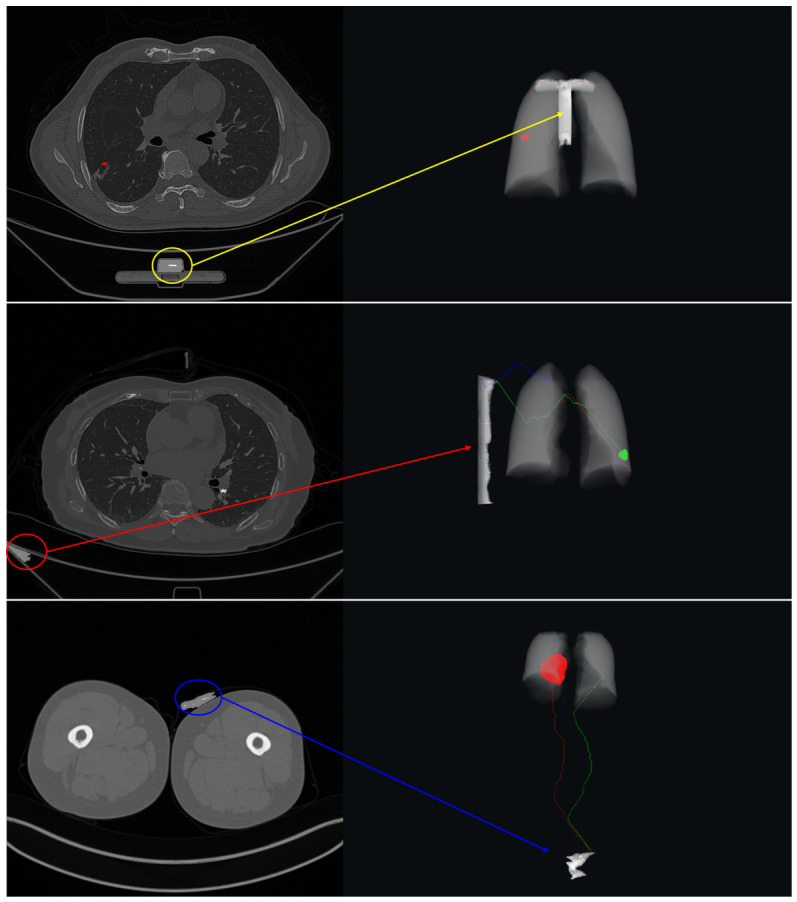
Three types of airway segmentation failure observed in the Lung-PET-CT-Dx dataset. For each row, the axial CT slice (left) and the resulting 3D path-planning output (right) are shown. (Top, yellow) Type 1: the central longitudinal air channel of a Philips scanner bed is misclassified as the trachea (case *Dx-A0083_FBP_338467*; Philips, *Chest 3D IMR*). (Middle, red) Type 2: the tapered lateral edge of the scanner bed is misclassified as a bronchial segment (case *Dx-A0084_IMR_575314*; Philips, *Chest 3D IMR*). (Bottom, blue) Type 3: in a Siemens whole-body acquisition (*CT WB 1.0 B30f*, 677 slices), the air gap between the patient’s thigh skin and clothing is misidentified as airway lumen (case *Dx-A0220_CT_WB_1_0_B30f_160816*).
